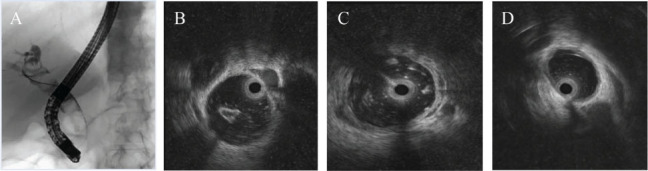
Female patient, 61 years old, with choledocholithiasis, who underwent ERCP combined with EST for stone extraction. (A) ERCP showed multiple stone shadows with diameters of 10–14 mm in the whole section of the choledocholithiasis (arrows). (B, C) ERCP combined with EST, no stone was seen on imaging after stone removal, and IDUS showed 2 <3 mm diameter stones in the middle part of the CBD (arrows). (D) Repeat extraction after lithotripsy, IDUS shows that the lower part of the CBD is ≥3 mm in diameter, and the residual stone has been removed. CBD, common bile duct; ERCP, endoscopic retrograde cholangiopancreatography; EST, endoscopic sphincterotomy; IDUS, intraductal ultrasound.
